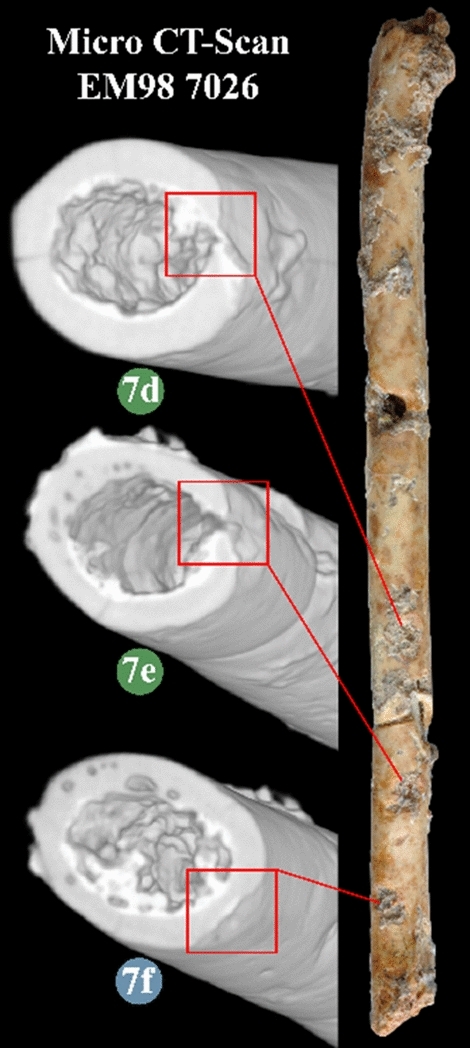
Mico CT-Scan cross-section of three worked areas covered with concretion on the complete aerophone (EM98 7026) showing that 7d and 7e are perforations and 7f. a shallow notch. (CAD and photos L.D.)*.*
